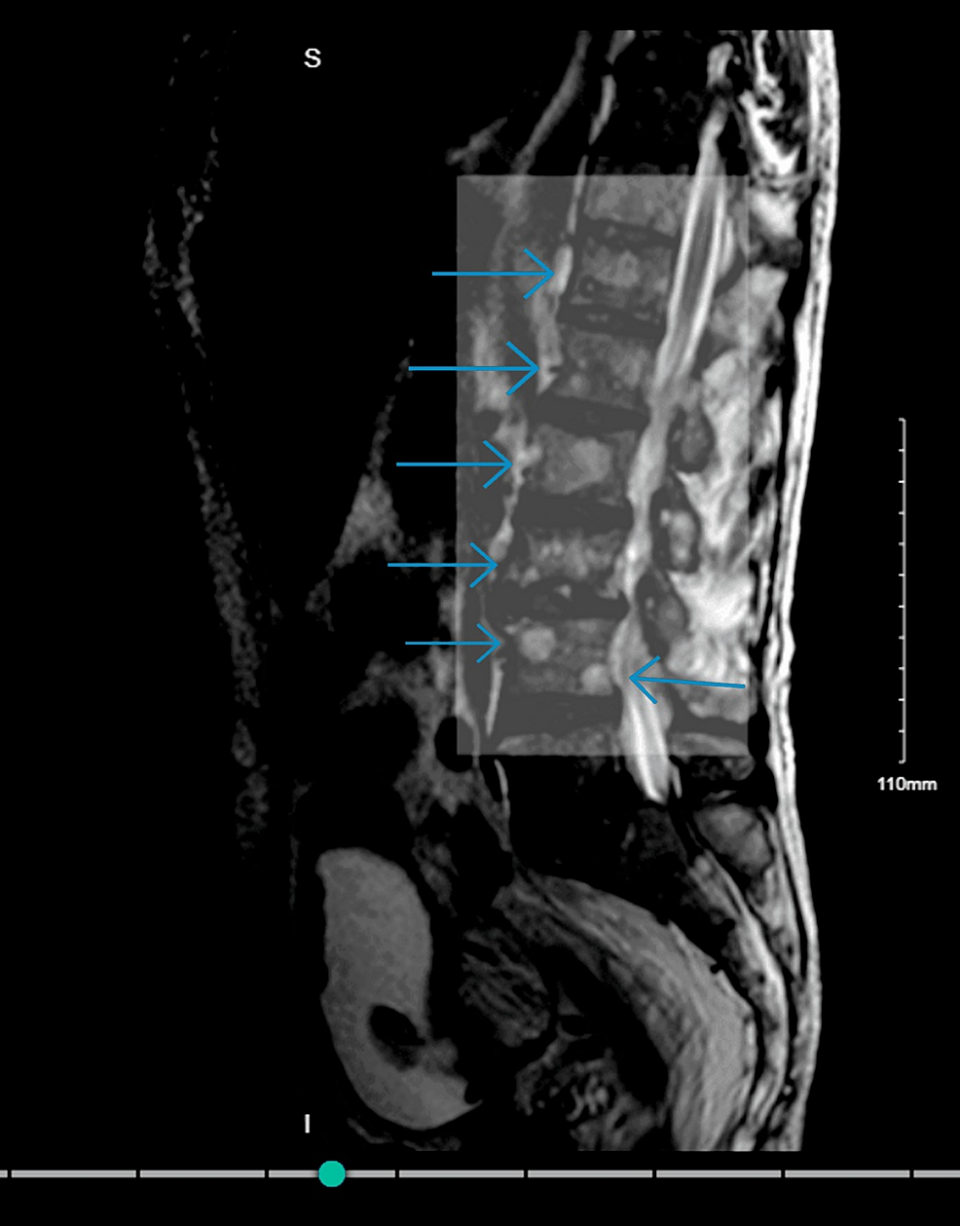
Magnetic resonance imaging of the spine shows multiple metastatic lesions (highlighted area)
